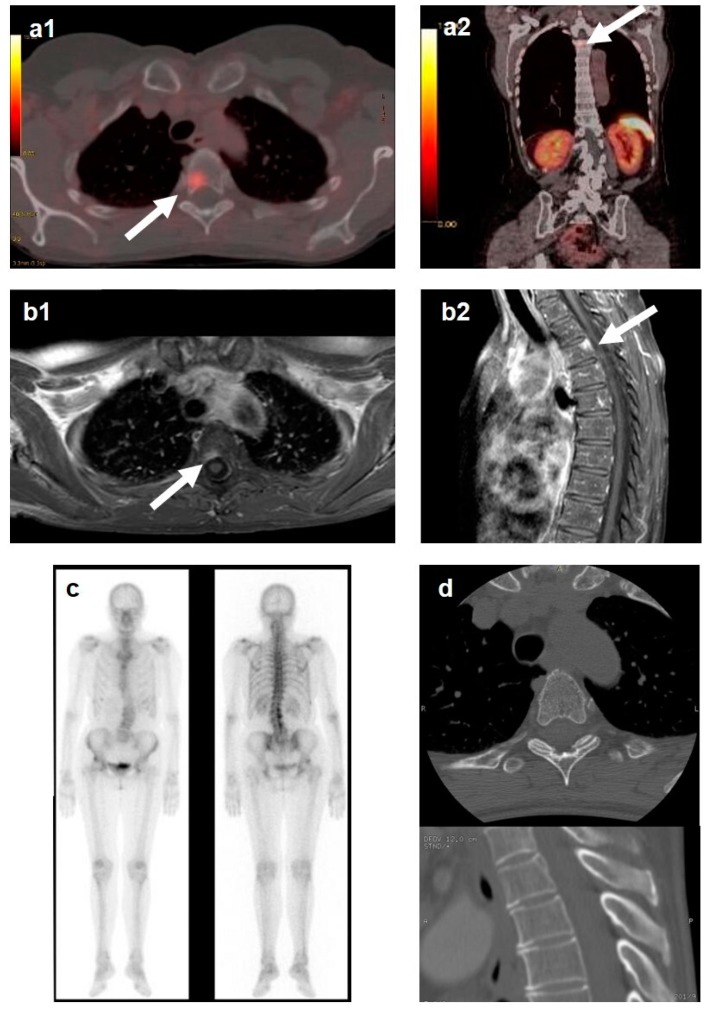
Thoracic vertebrae (T4) bone metastasis (BM) was diagnosed two years after a liver metastasis in a 66-year-old woman with a history of ileal neuroendocrine neoplasm. Somatostatin receptor scintigraphy (SRS) was performed twice in the last four years of follow-up and did not detect BM. (a1,a2) T4 vertebra BM was first detected by 68Ga-DOTATOC positron emission tomography (PET)/ computed tomography (CT); (b1,b2) BM was confirmed by magnetic resonance imaging (MRI); (c) 99mTc-bone scintigraphy did not detect BM; (d) computed tomography was also negative for BM. Arrow indicates the BM.
